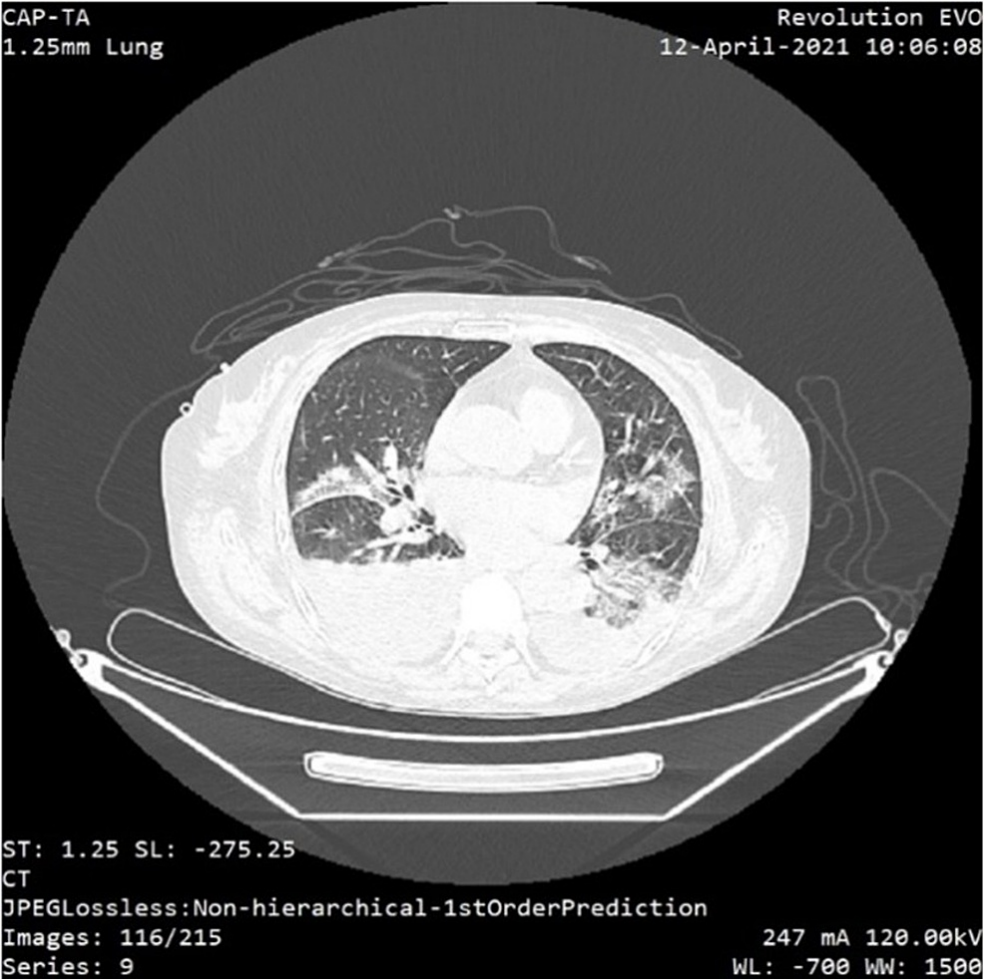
Thorax CT scan revealing moderate bilateral pleural effusions and typical glass opacities, peripheral distributed in approximately 50% of the lung parenchyma
